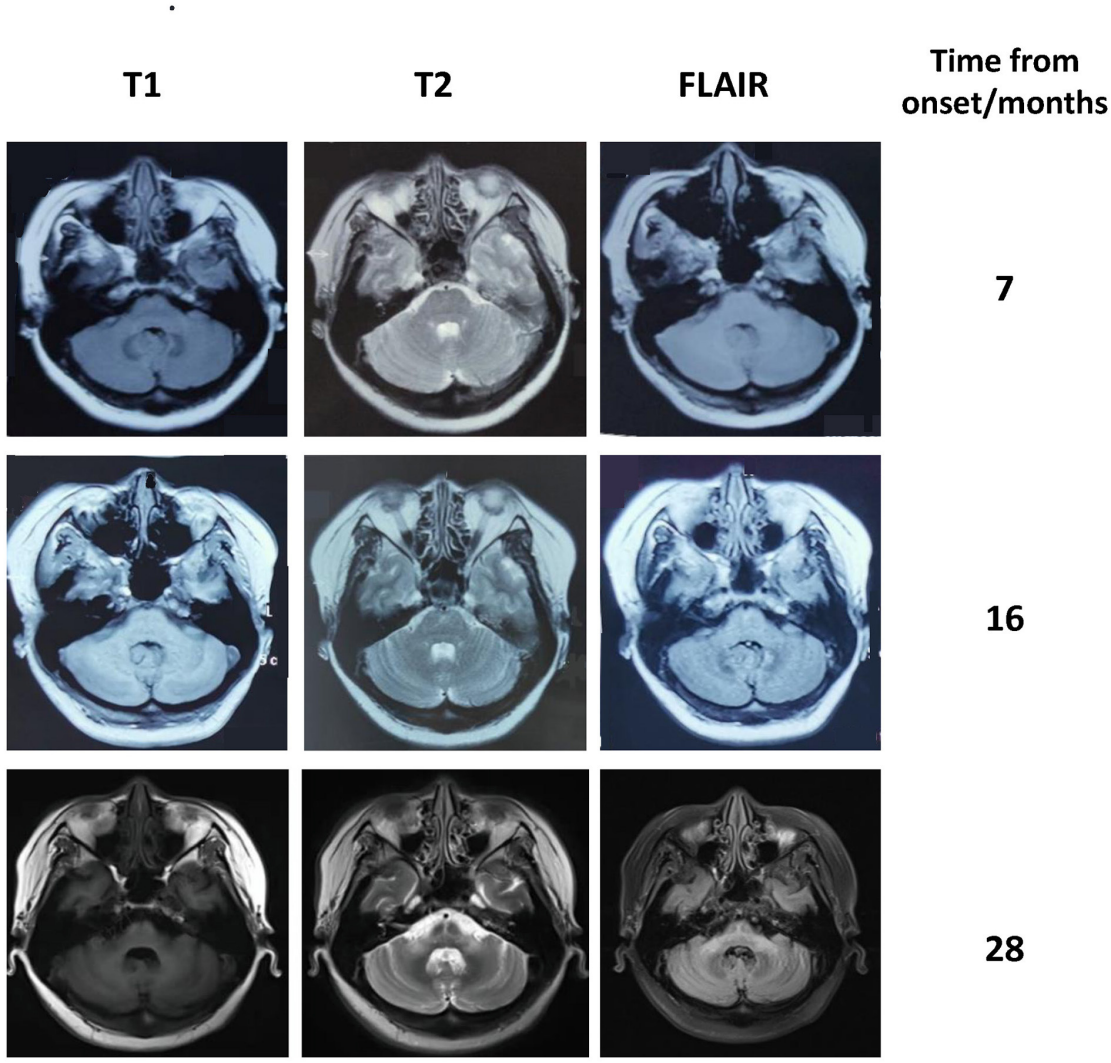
Brain MRI of our patient showing progressive atrophy of the cerebellum and the middle cerebellar peduncle. The hot cross bun sign and the abnormal signal in the middle cerebellar peduncles became more pronounced over time.
